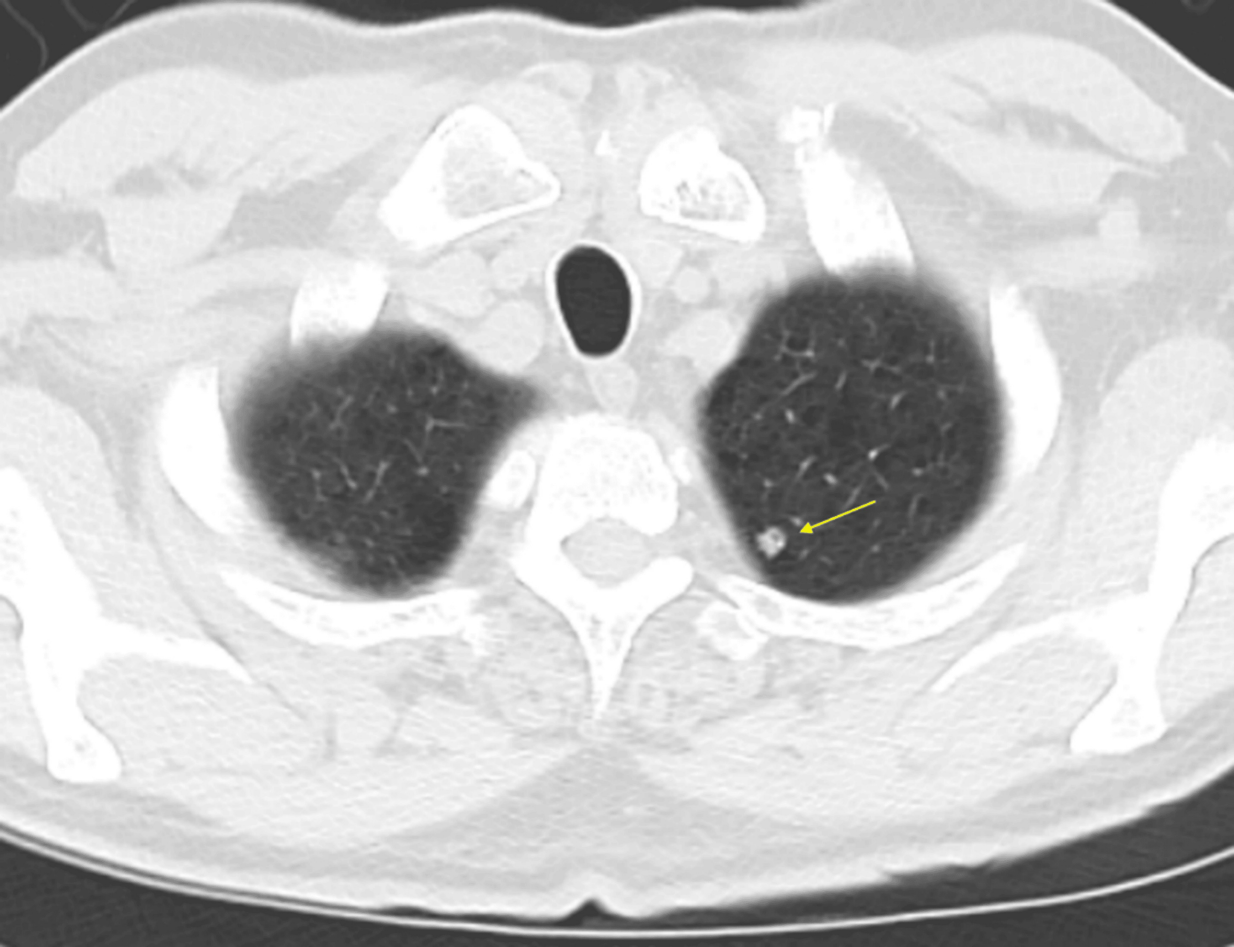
Chest CT scan in October 2023 showing a 7 x 5 mm nodule in the upper lobe of the left lung CT: computed tomography
